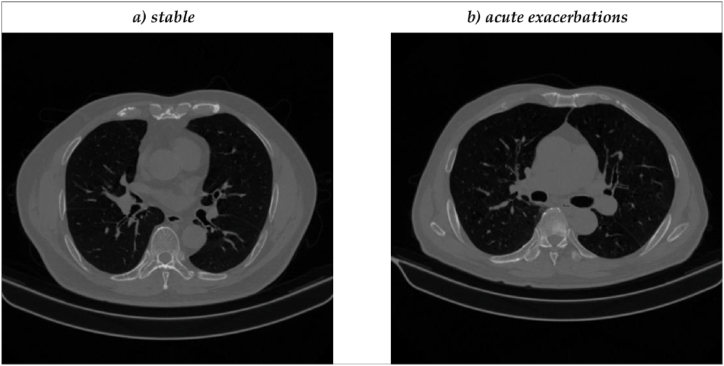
The inspiratory and expiratory low-dose CT images of two patients who stabilized in the second year and experienced an acute exacerbation. a) The CT image of a stable COPD patient. b) The CT image of an AECOPD patient.
